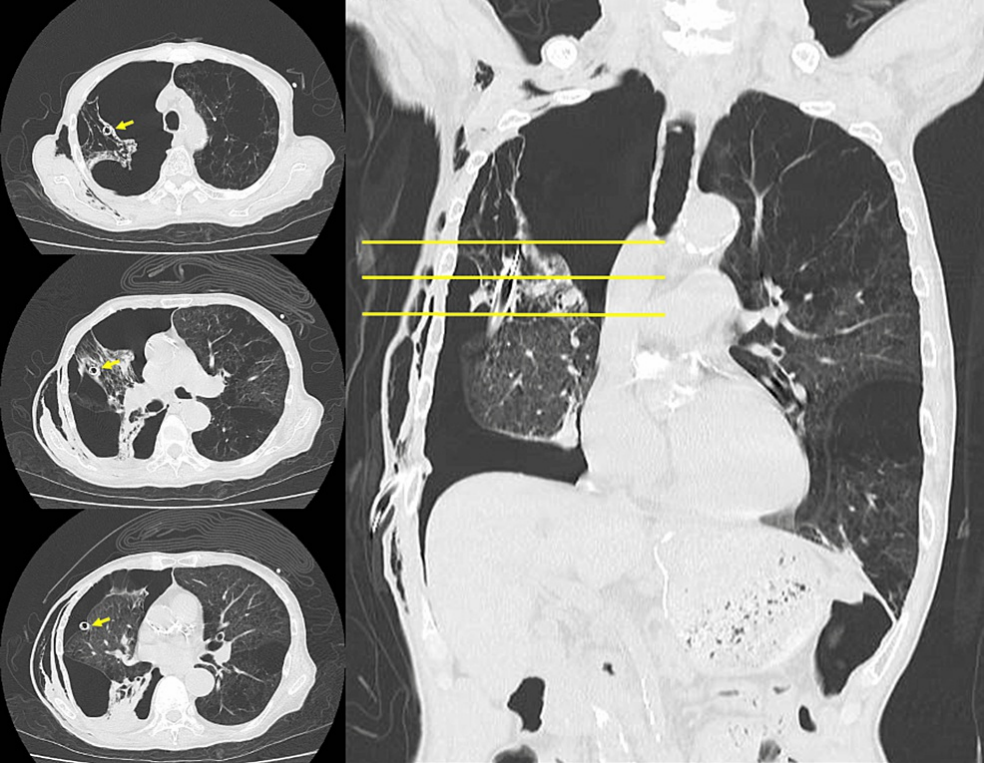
Computed tomography taken a day after chest tube insertion The chest tube was inserted 6 cm into the right upper lobe (yellow arrows), and lung collapse was worse than it was before the chest tube insertion. The images to the left of the coronal section are axial images at the level of the yellow lines.
